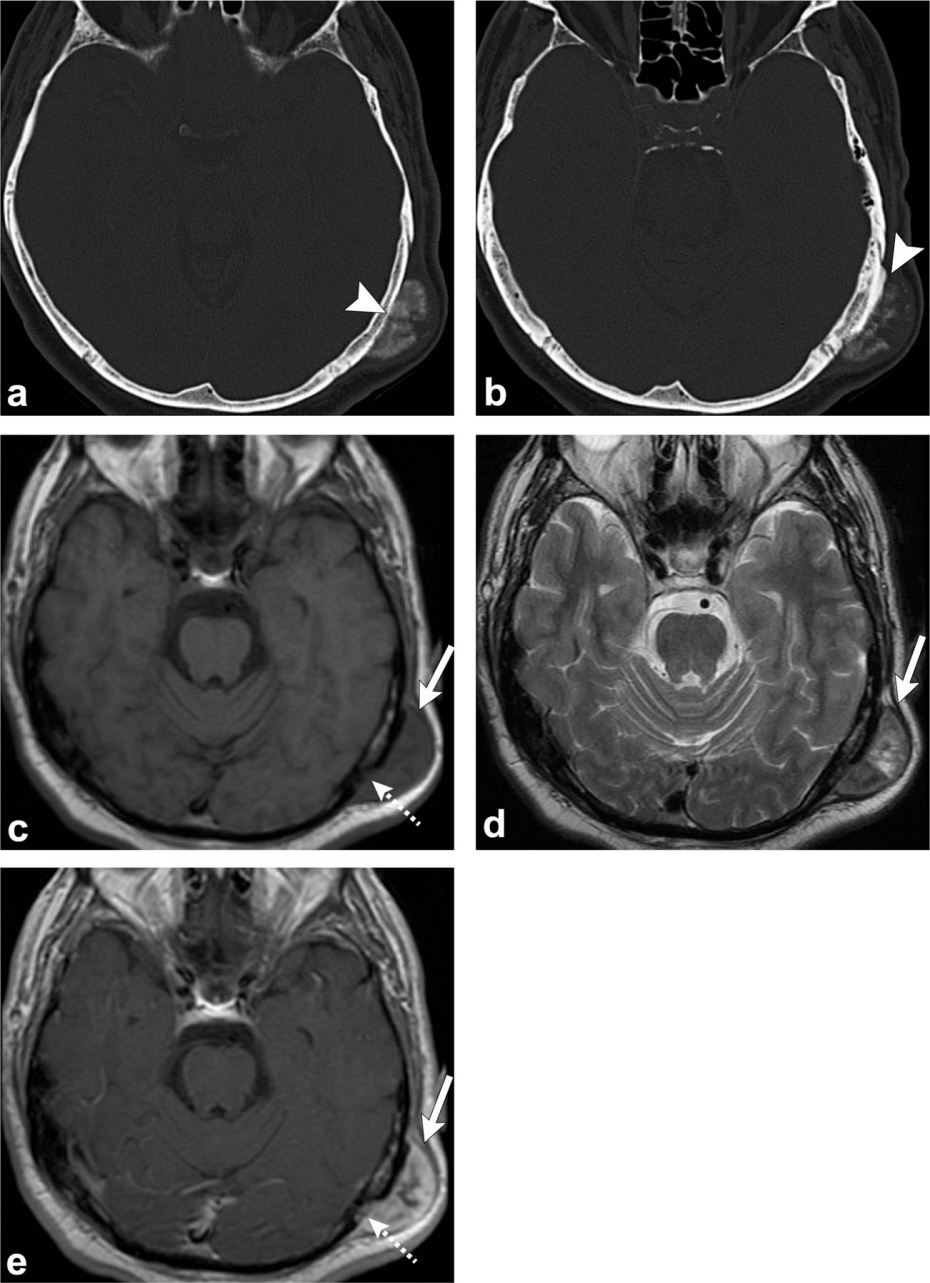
Parosteal osteosarcoma. Axial CT (a, b) images show a lobulated exophytic mass (*arrowheads*) with central dense ossification in the left temporo-occipital region. Axial T1-weighted (c), axial T2-weighted (d) and axial post-contrast T1-weighted (e) images re-demonstrate a juxta-cortical enhancing lesion abutting the outer table of the left temporo-occipital bones (*arrows*) with a small component extending into the medullary cavity with associated cortical disruption (*dashed arrows*)
